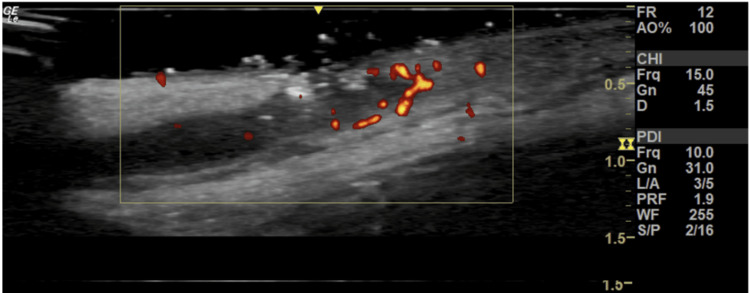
Hidradenitis suppurative ultrasound image showing hypoechoic fistulous tract with hyperechoic fragments, with increased vascularity observed on Doppler.
